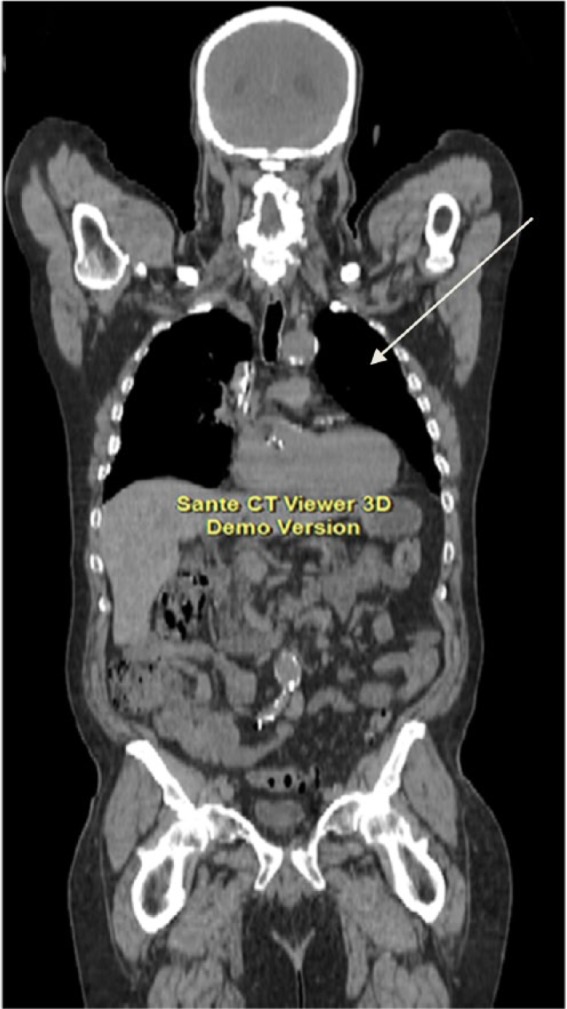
June 2015, CT scan, showing absence of the left pulmonary metastatic
